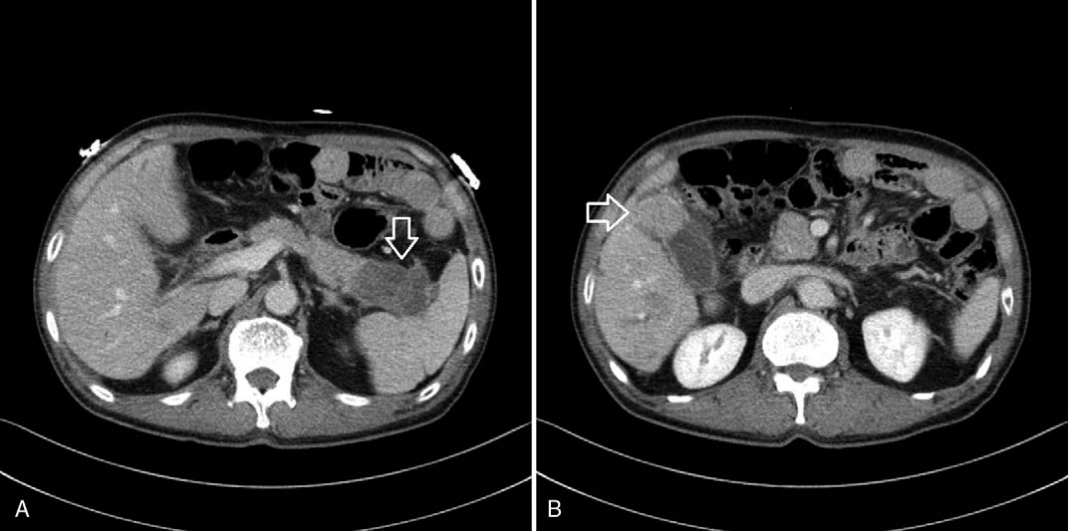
Abdominal CT. (A) A 63 × 37 mm heterogeneous enhancing low-attenuating mass in the tail of the pancreas. (B) A 40 × 35 mm heterogeneous enhancing mass in the gallbladder fundus (arrows). CT = computed tomography.
